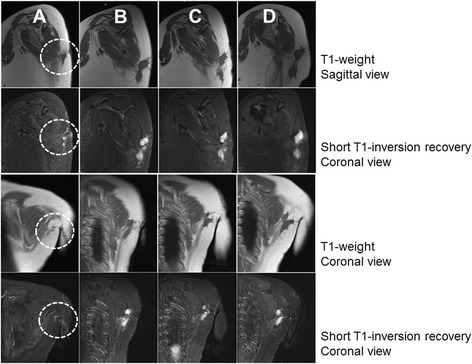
Representative MRI from case No. 3. Column a: sixth month after first surgical operation and three months before starting of the procedure. Column b: just prior to the first ILSI determined progression of tumor. Column c: tumor stabilization from baseline after the final protocol of ILSI. Column d: progression disease from baseline after the third month of ILSI
